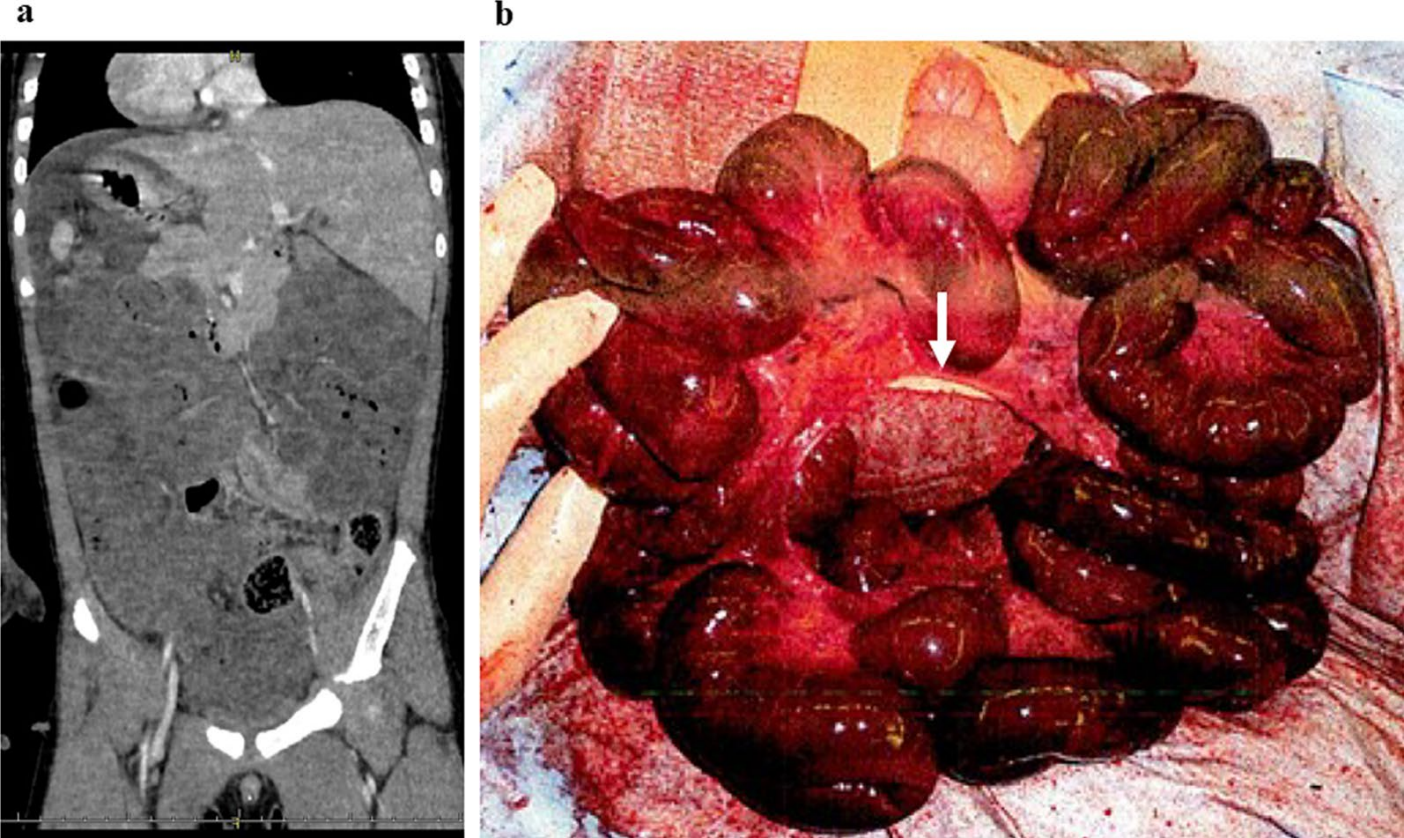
Preoperative CT and intraoperative findings. a Preoperative CT: a poor contrast effect on the small intestine. b Intraoperative findings: strangulated bowel obstruction caused by an intestinal mesenteric hiatal hernia. White arrow: intestinal mesenteric hiatal hernia
